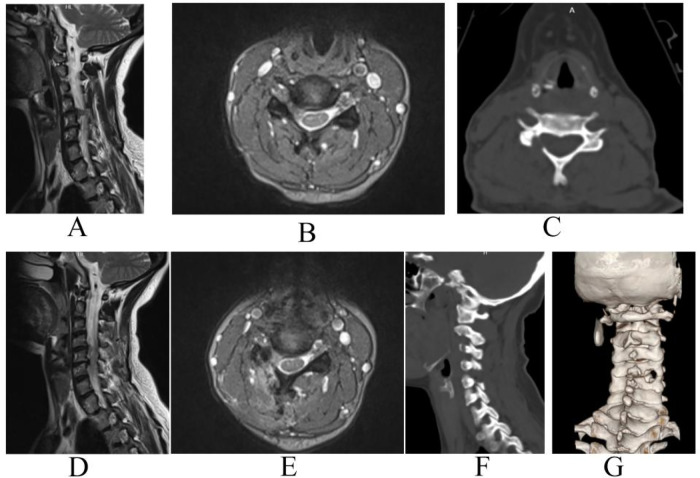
A 56-year-old female patient with C4/5 right cervical disc herniation who underwent UBE keyhole foraminotomy. (A) Sagittal view of MRI preoperatively; (B) transverse section view of MRI preoperatively; (C) transverse section view of CT postoperatively; (D) sagittal view of MRI postoperatively; (E) transverse section view of MRI postoperatively; (F) sagittal view of CT postoperatively; (G) 3D reconstruction of cervical spine postoperatively.
